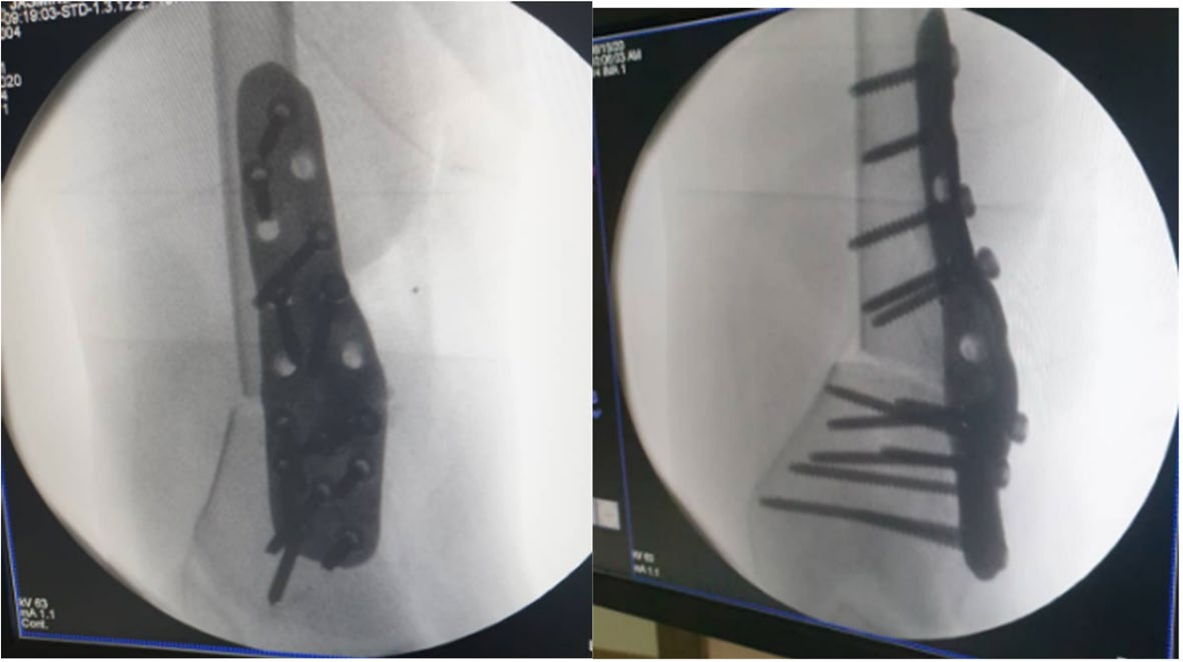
X-ray image of implant positioning right after surgery showing excellent fit
